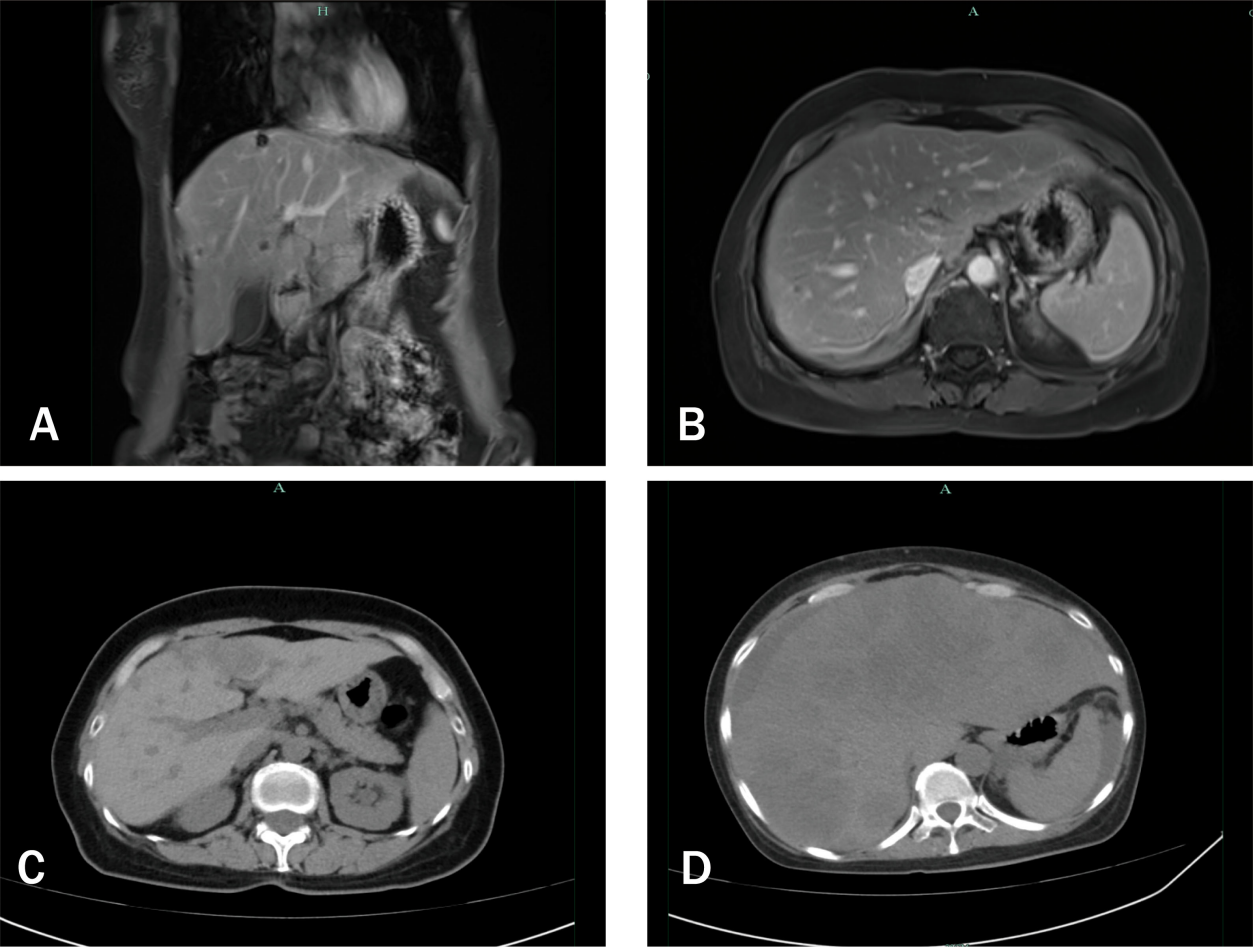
Serial imaging of hepatic metastases. (A, B) Contrast-enhanced T1-weighted MRI (13 June 2025) showing sustained reduction in intrahepatic tumor burden. (C) Contrast-enhanced CT (16 August 2025) demonstrating early regrowth of liver metastases. (D) CT (8 November 2025) revealing diffuse, multifocal hepatic progression consistent with HVC.
